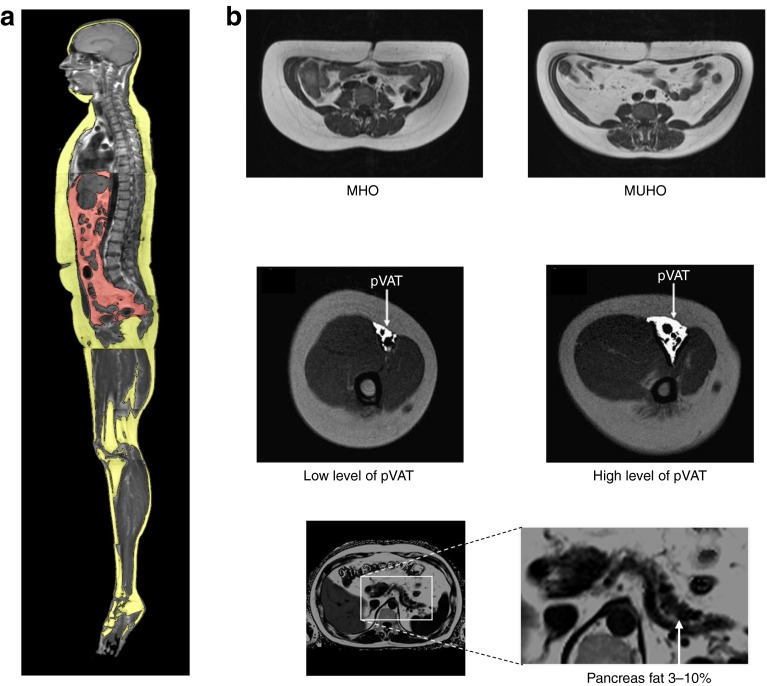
Subphenotypes of obesity. Whole-body MRI measurements are used to quantify fat compartments [52–58]. (a) Yellow, subcutaneous adipose tissue; red, visceral adipose tissue. (b) pVAT, perivascular visceral adipose tissue
